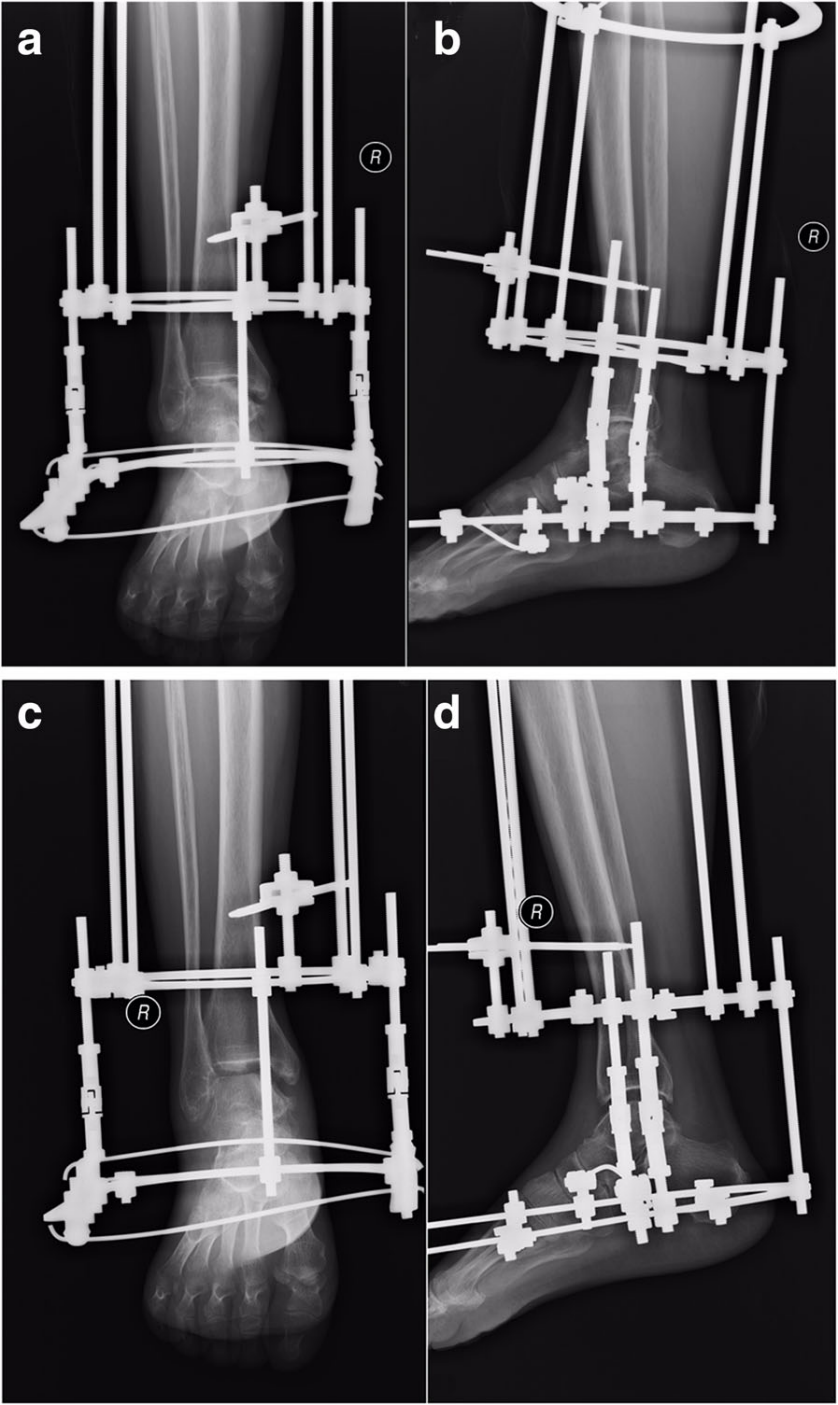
The radiographic views of the ankle before and after distraction. a, b The anteroposterior and lateral radiographs taken 1 day postoperatively showed the Ilizarov fixator was fixed appropriately. c, d The anteroposterior and lateral radiographs taken 1 week postoperatively showed joint space significantly enlarged due to 0.5-mm-distraction arthroplasty twice daily for five consecutive days
